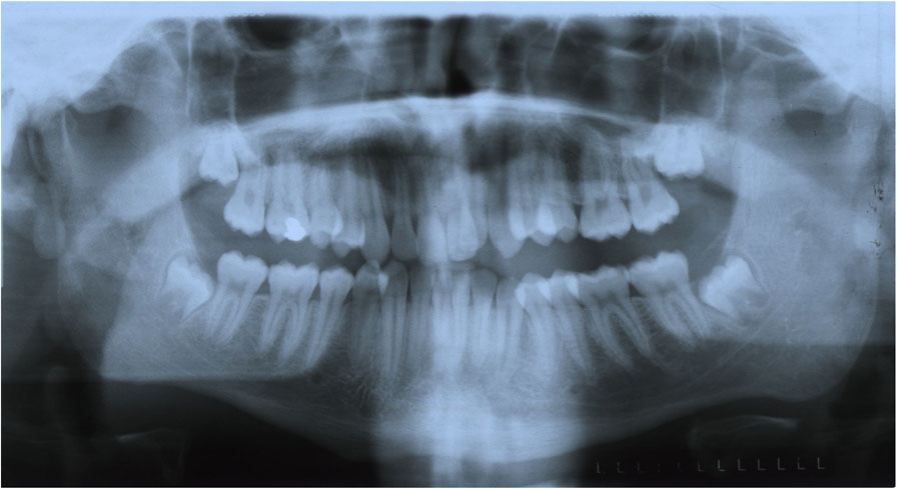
Presurgical orthopantomograph
